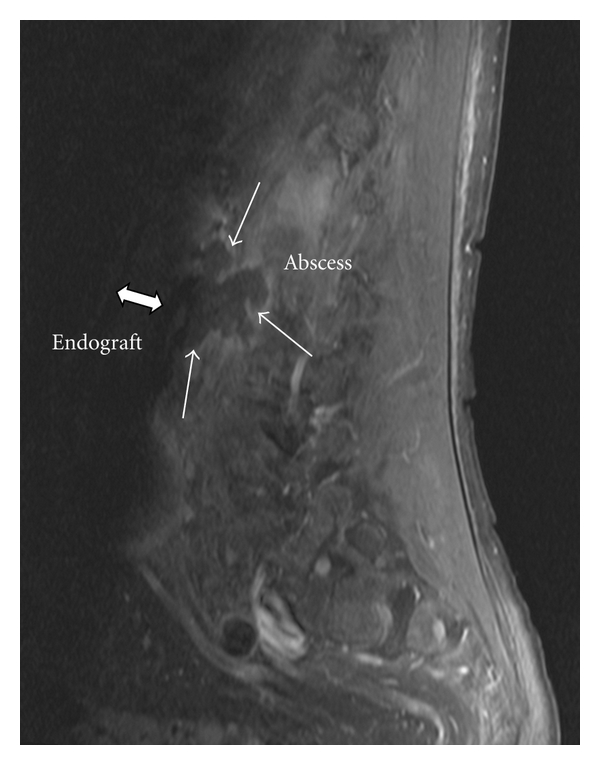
Sagital view of preoperative MRI-scan showing a retroaortal fluid collection with slight enhancement reaching to the dorsal part of the prosthetic endograft.
